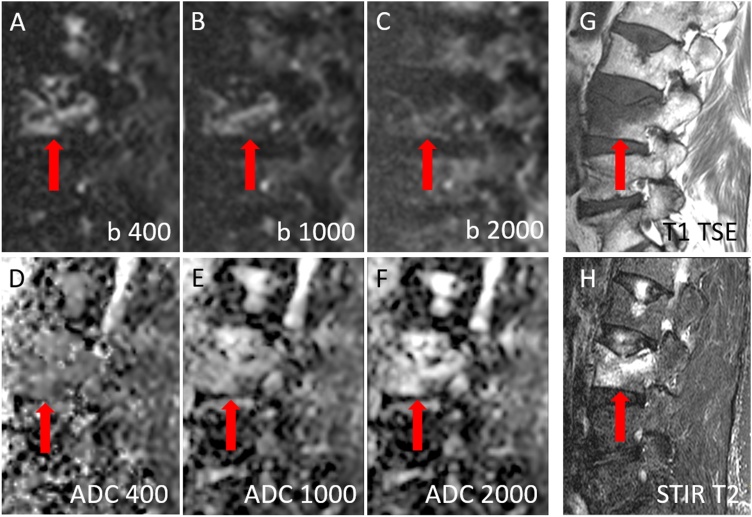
69 years old female patient with osteoporotic vertebral body fracture of lumbar vertebra L3 (red arrow). Hyperintense signal of fractures relative to normal vertebral bodies on DWI with b400 (Fig. 2A) and on DWI with b1000 (Fig. 2B), but no hyperintensity on DWI with b2000 (Fig. 2C). Moderate to high signal intensity on ADC of b = 0,400 (Fig. 2D), on ADC of b = 0,1000 (Fig. 2E) and on ADC of b = 0,2000 images (Fig. 2F). The fracture is hypointense on T1w TSE images (Fig. 2G) and inhomogeneously hyperintense on STIR T2w images (Fig. 2H) (For interpretation of the references to colour in this figure legend, the reader is referred to the web version of this article).
